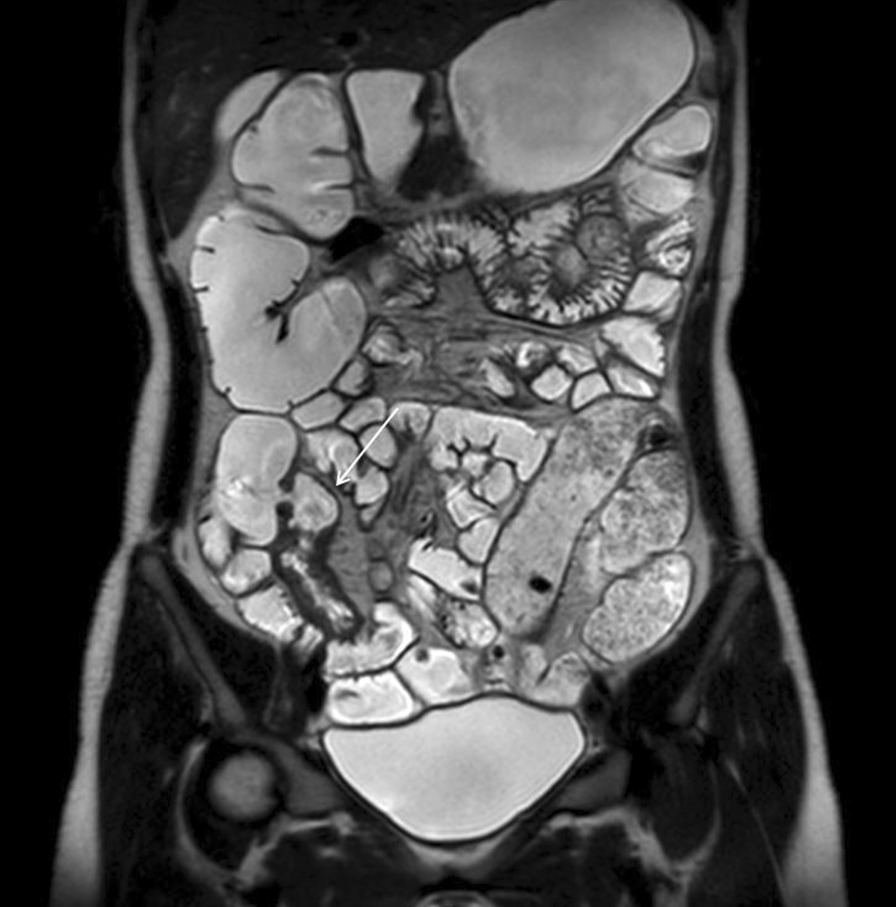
MR enterography. Coronal view of the patient’s MRI enterography with segmental and uneven wall thickening of ulcerative lesions in distal to terminal ileum (arrow)
